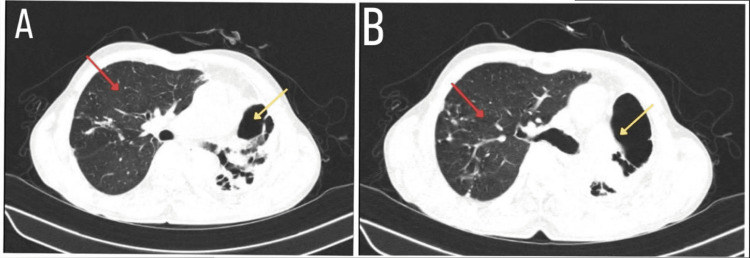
The HRCT shows a destroyed left lung (yellow arrow) and a compensatory hyperinflated right lung (red arrow) in both A and B. HRCT: high-resolution computed tomography
